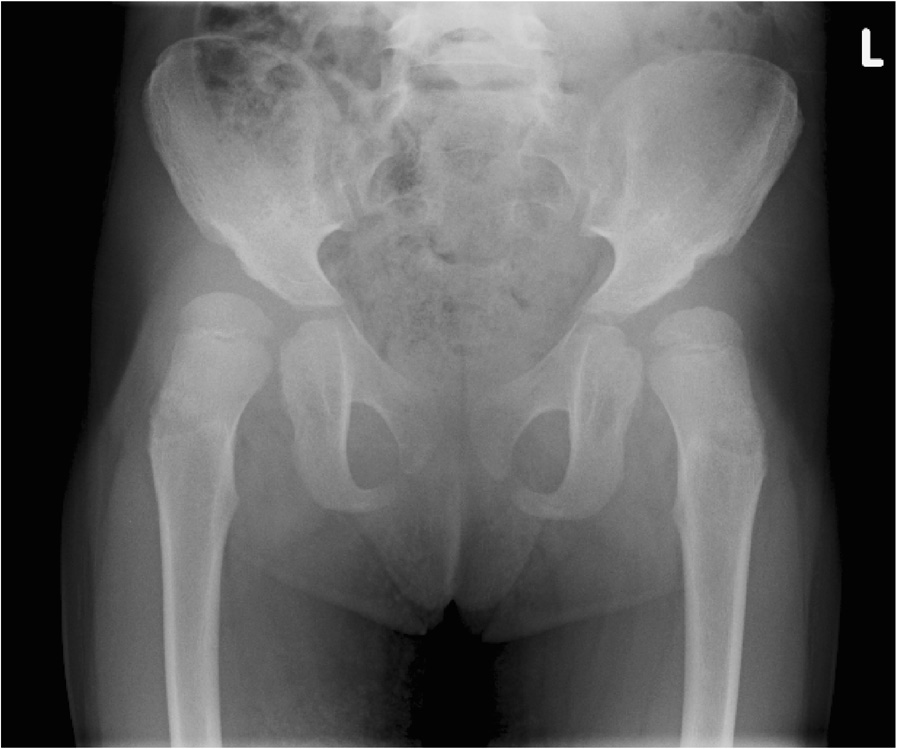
Pelvic X-ray at age 4 years.
